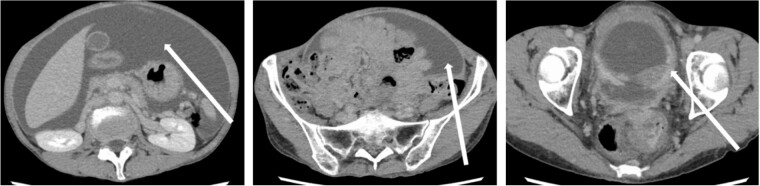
CT findings. In addition to significant ascites accumulation, there was a partial bowel obstruction-like finding.
